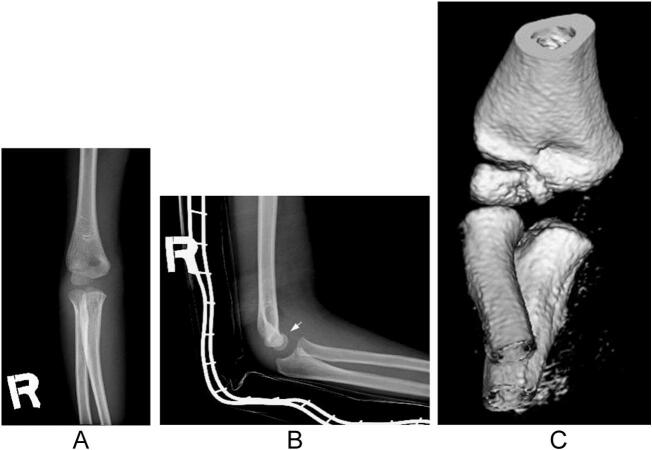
Simple X-ray and computed tomography (CT) images of the right elbow show an avulsion fracture of the capitellum. (a) Anteroposterior view. (b) Lateral view. The arrow indicates the avulsion fragment. (c) Three-dimensional anterior view.
